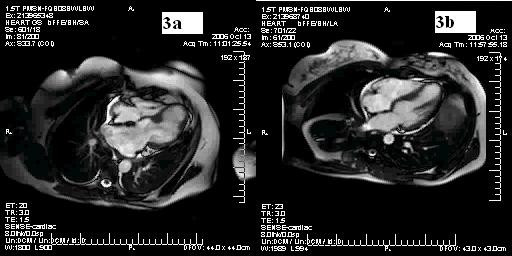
Cardiac Magnetic Resonance Images. 3a) Cardiac magnetic resonance image (MRI) showing midventricular systolic thickening and 'hourglass' left ventricular shape in case index-2 patient and her daughter case-index 3(3b). Note the formation of apical aneurysms in both cases.
